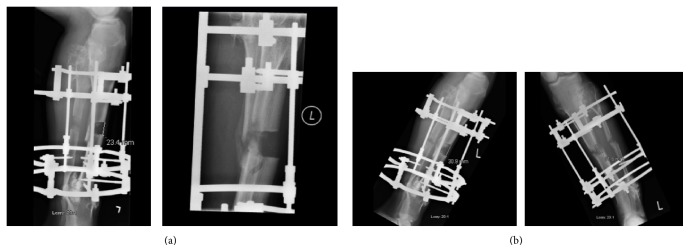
(a) Anteroposterior (on the right) and lateral radiographic (on the left) views of left tibia (case 4) showing very discrete bone regenerate after 6 weeks of distraction at a rate of 0.25 mm × 2 times/day. (b) Anteroposterior (on the right) and lateral radiographic (on the left) views of left tibia (case 4) after 7 weeks of the accordion technique showing significantly improved osteoformation.
